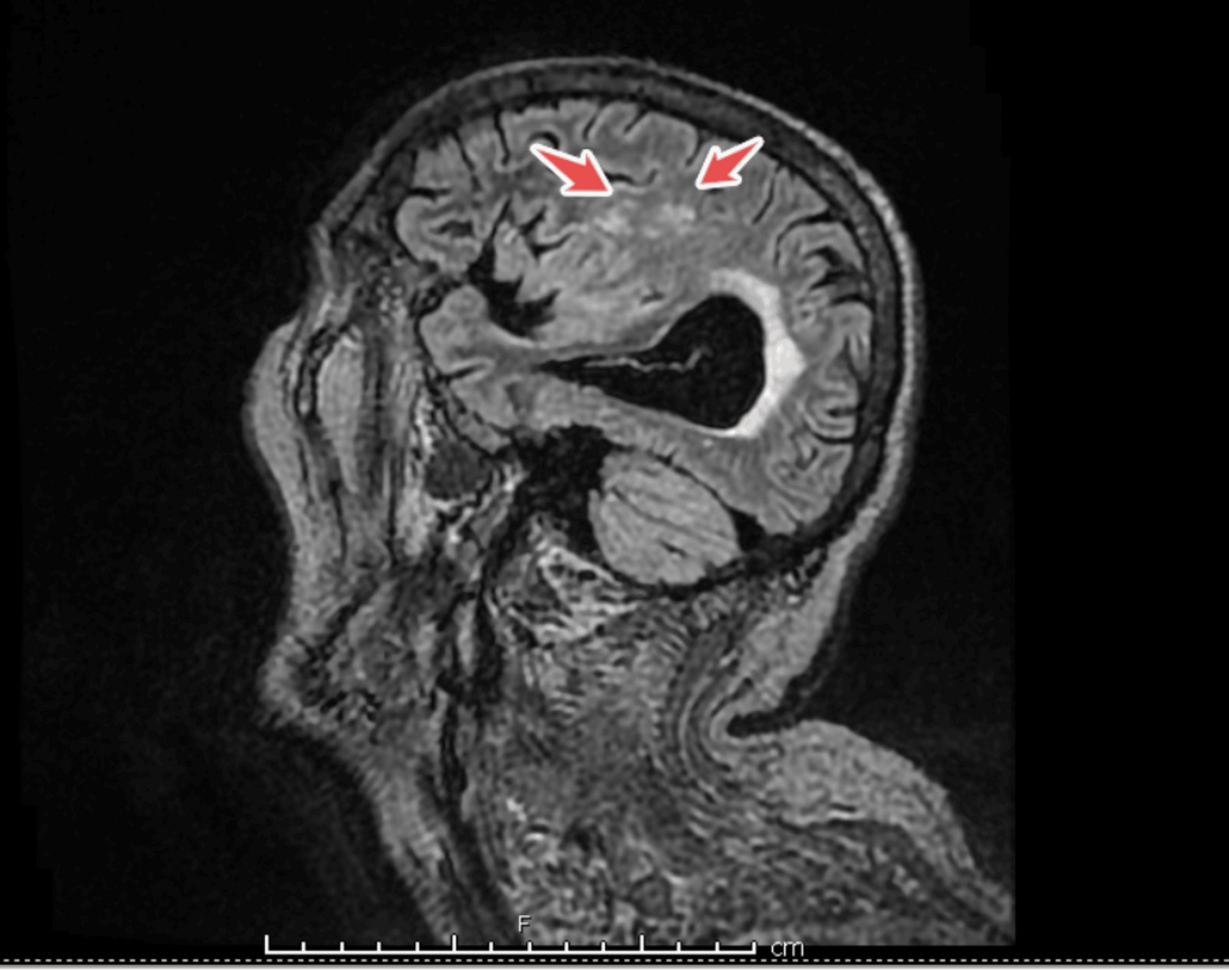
Sagittal MRI of the brain showing hyperintense lesions (red arrows), consistent with septic embolic lesions secondary to infective endocarditis MRI, magnetic resonance imaging
